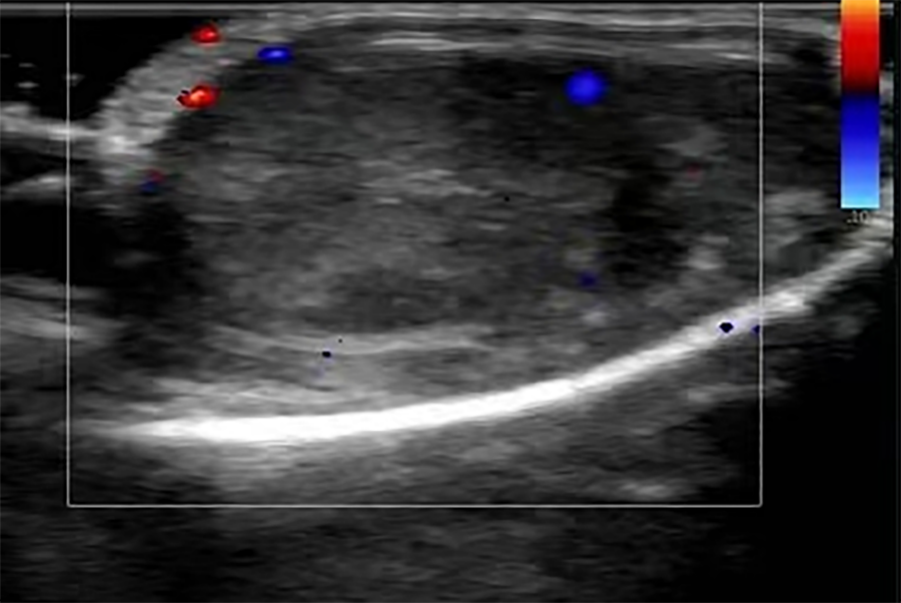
Ultrasound image of the right testis on hospital admission. The color Doppler ultrasound image revealed the uneven echo of the right testis, which showed no significant blood flow signal.
